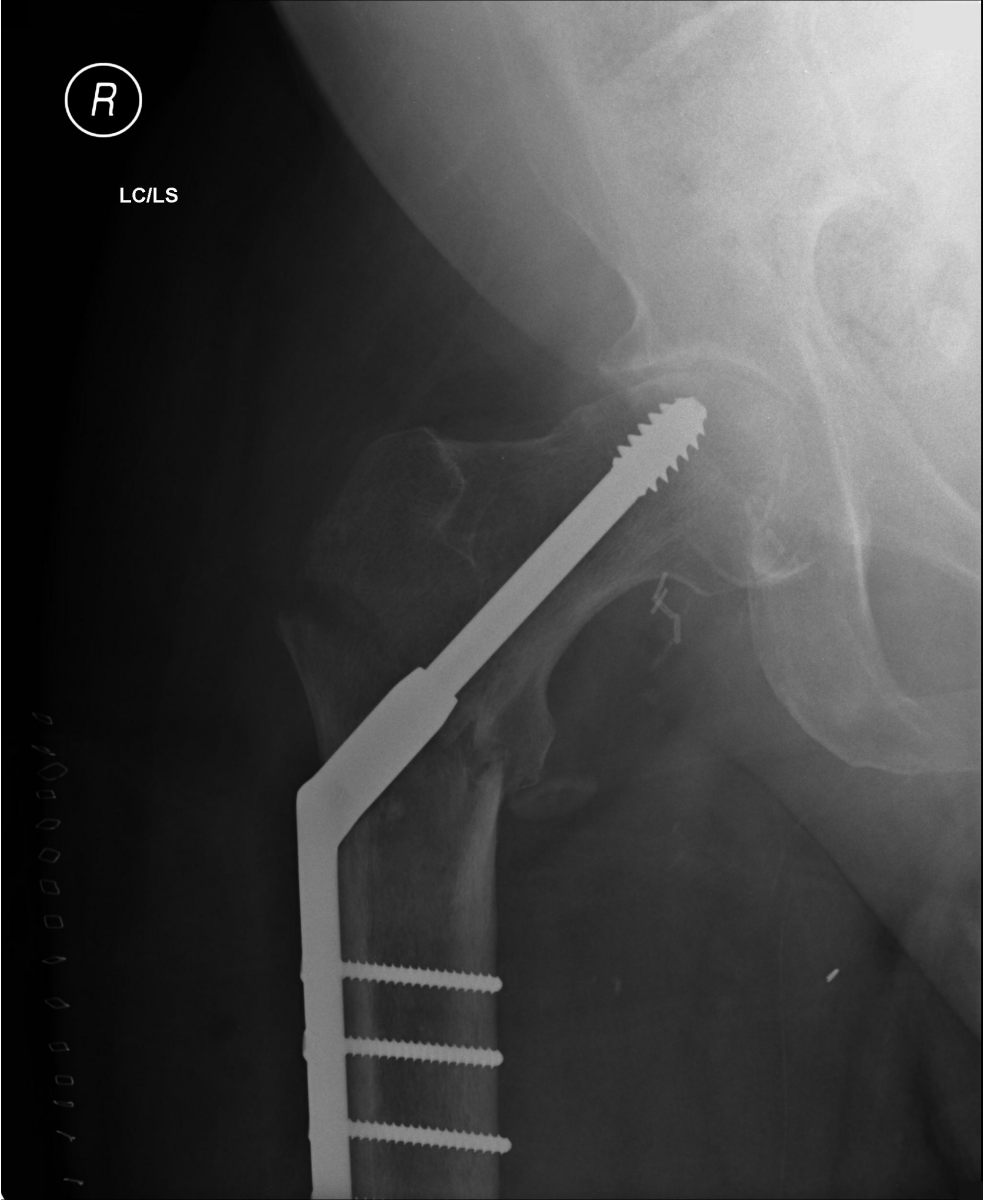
Postoperative plain radiograph of the pelvis showing fracture fixation with a dynamic hip screw.
